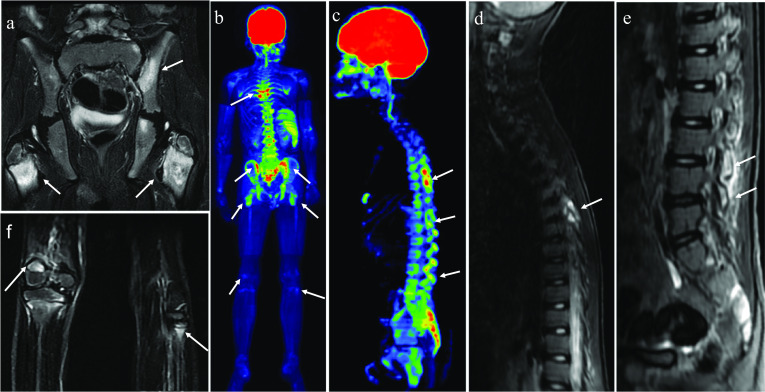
10-year-old child with CNO and a history of intermittent hip pain since he was 4 years old with elevated markers of inflammation under investigation for juvenile idiopathic arthritis. MRI of the pelvis showed bone marrow edema in the intertrochanteric periphyseal regions and in the sacroiliac joint (a), which was suggestive of CNO. WB-MRI was performed on the same day (b–f). Functional diffusion-weighted imaging (DWI) sequences (b and c) detected multiple lesions in the posterior elements of the thoracolumbar spine, sacroiliac joints and intertrochanteric regions, in addition to the distal metaphysis of the right femur and proximal metaphysis of the left fibula. The lesions are demonstrated in STIR morphological sequences as signal change with an edema pattern in the corresponding locations (d, e and f). HLA B27 was positive
